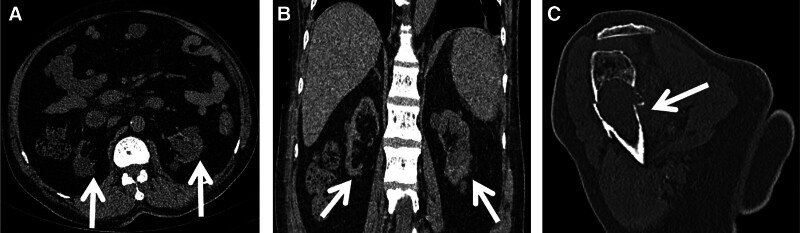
A 58-year-old patient with end-stage renal disease (ESRD) experiencing discomfort and pain in the left shoulder for the past month. CT scans in the axial (A) and coronal (B) planes from a year ago depict numerous cysts (>3). Recent coronal (C) CT imaging indicates bone destruction and a fracture in the proximal left humerus. CT = computed tomography.
